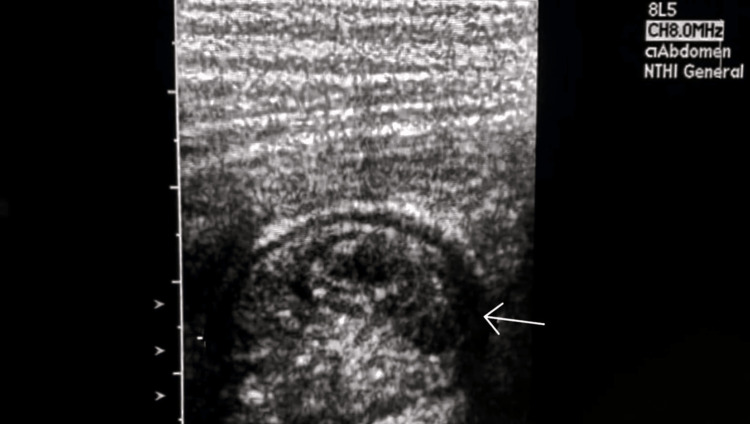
Intussusception - image 2 Cross-sectional 5-8 MHz ultrasound image obtained over the right lumbar region demonstrating the target sign
